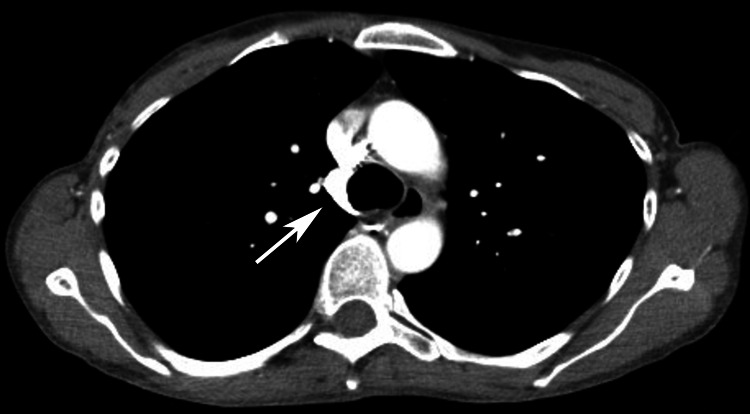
CT of the chest with IV contrast obtained for unrelated reasons one year prior to injury showing no evidence of pseudoaneurysm.
